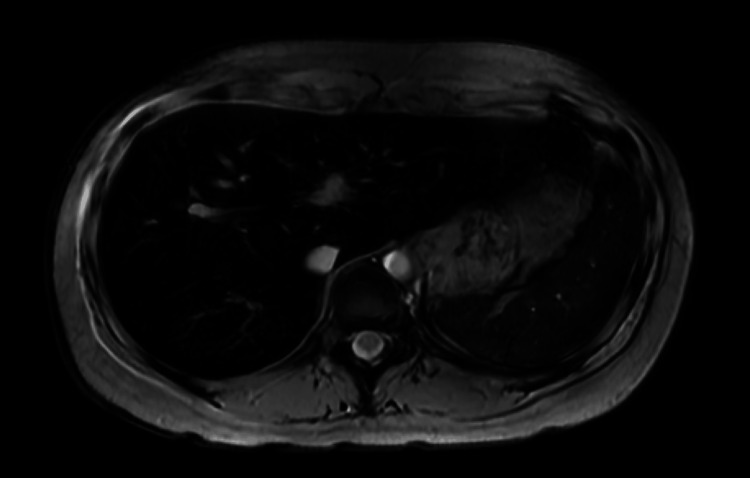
Liver MRI T2*-weighted image: the liver and spleen are relatively more sensitive to magnetic substances such as iron in the tissues than the muscle and bone marrow. The diagnosis reached was iron overload secondary to the oral administration of amino acid chelated iron (bisglycinate chelate iron, Ferrochel) MRI: magnetic resonance imaging
